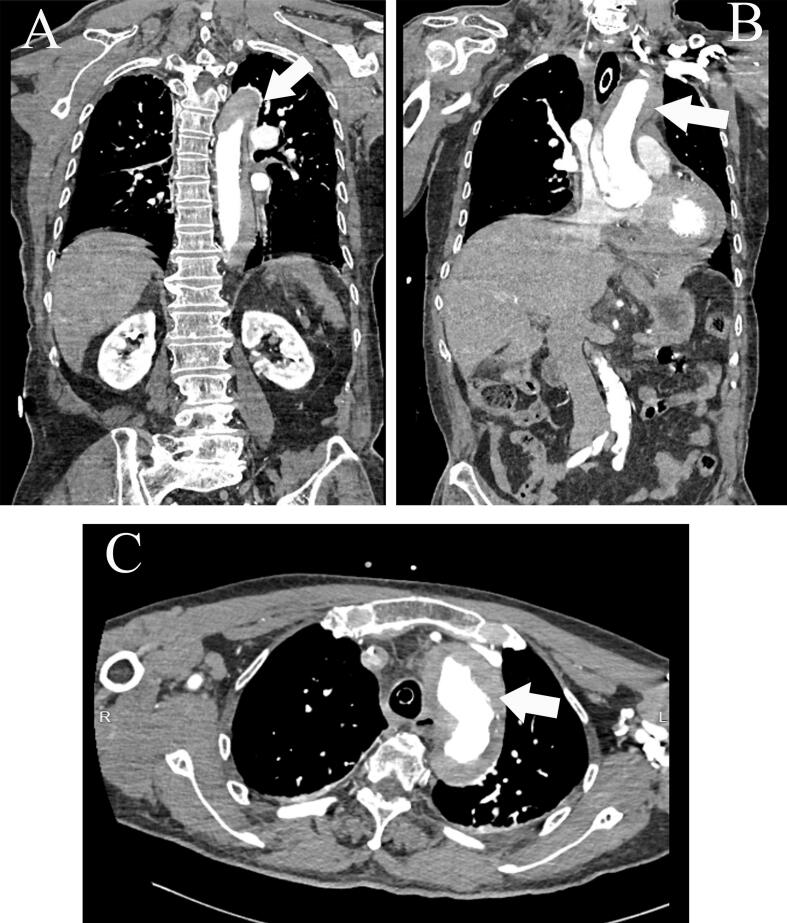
Aortic CTA confirmed Stanford Type A dissection extending from the aortic root to the L1 vertebral level.
